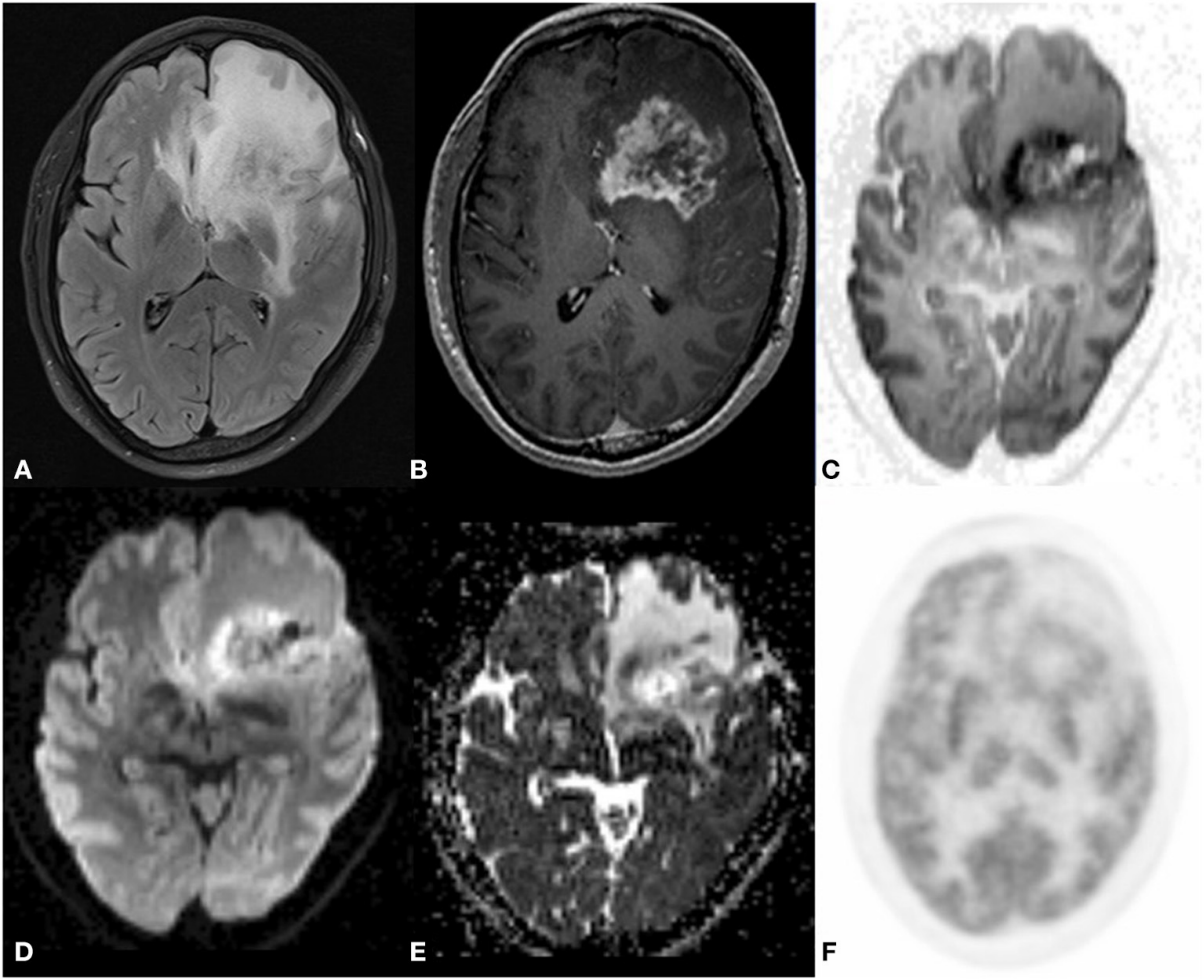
A 43 year old male postoperative case of Glioblastoma IDH mutant in the left frontal lobe, 5 year follow up MRI FDG PET axial images T2-FLAIR (A) post-contrast T1 (B) Inverted DWI (C) DWI b2000 (D) ADC (E) NAC PET (F) showed a T2 heterogeneous hypointense lesion with Swiss cheese and nodular enhancement and elevated perfusion. The lesion shows DWI restriction with hypermetabolism on PET. There is a match on the inverted DWI image and the NAC PET image. Suggestive of recurrence.
